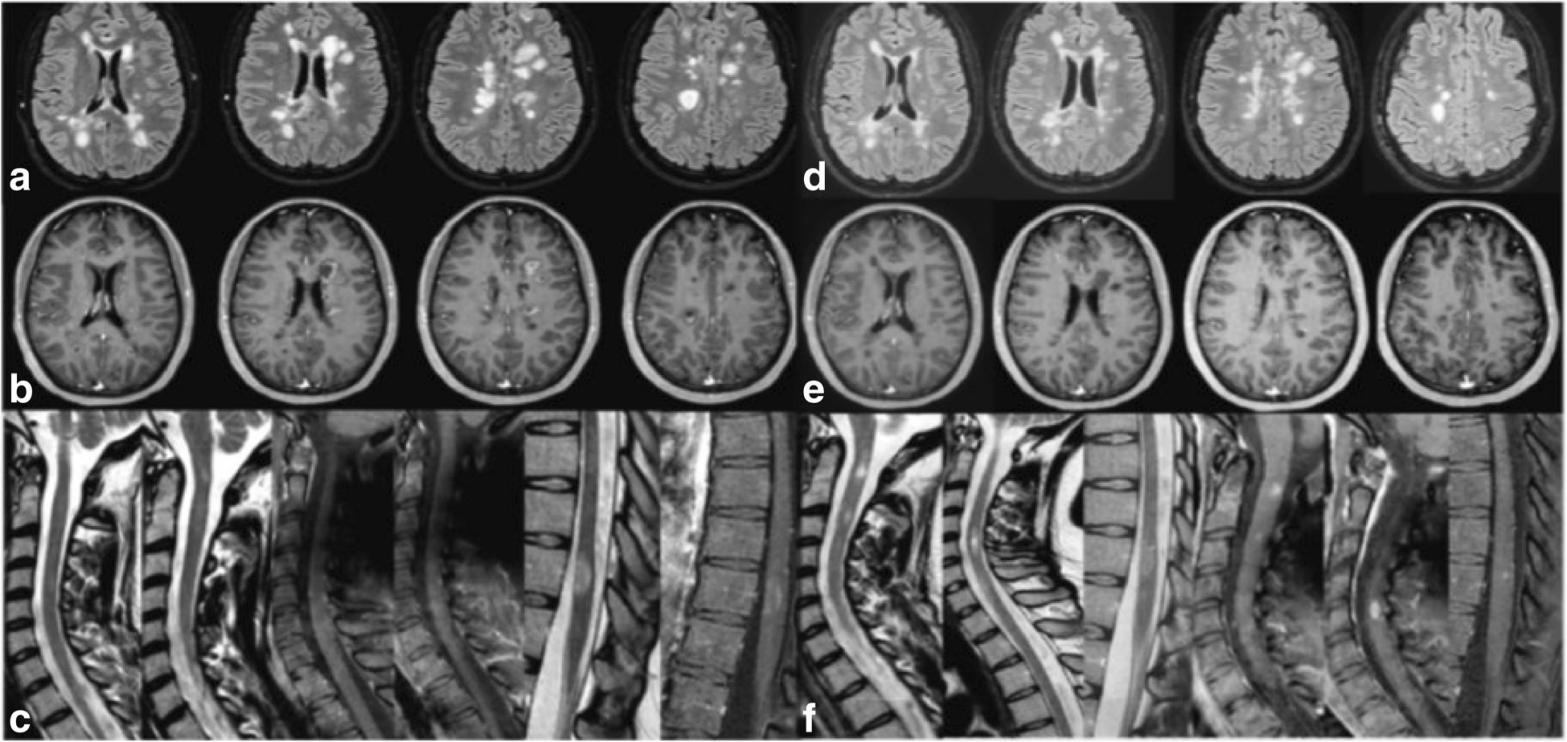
Axial (a) T2-weighted FLAIR MRI under clinical relapse showing multiple new supratentorial lesions; axial (b) T1-weighted images showing lesions with ring gadolinium-enhancement, (c) sagittal T2-weighted FLAIR MRI showing different spinal cord lesions, one of them with ring gadolinium-enhancement on T1-SPIR weighted image. (d), (e), (f) are the same images repeated after 40 days from high dose of steroids i.v.
